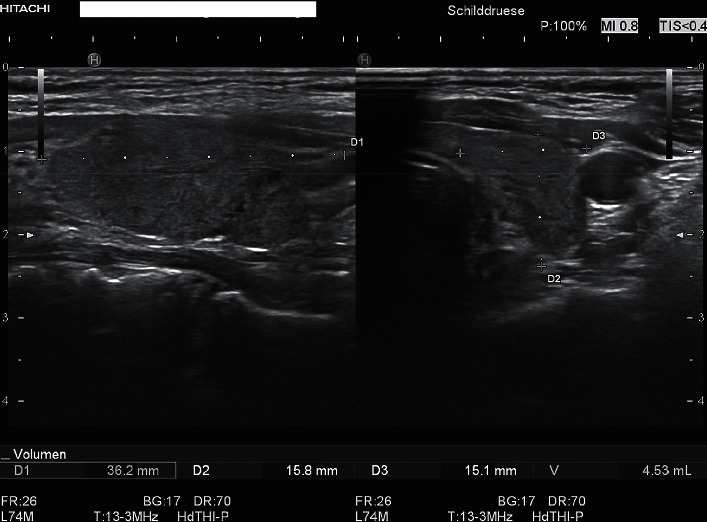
This ultrasound picture shows a hypoechogenic texture with pseudonodular lesions typical for SAT.
